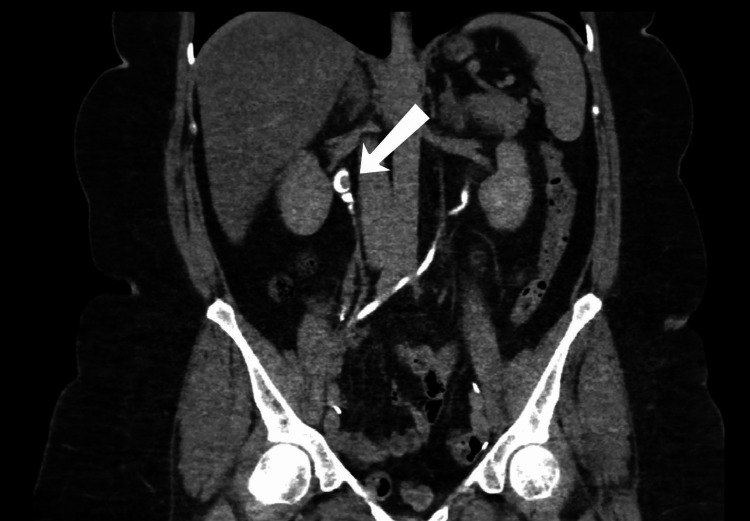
Pelvis and abdomen computed tomography with IV contrast showed a 7 mm enhancing lesion in the right proximal ureter. IV: intravenous
